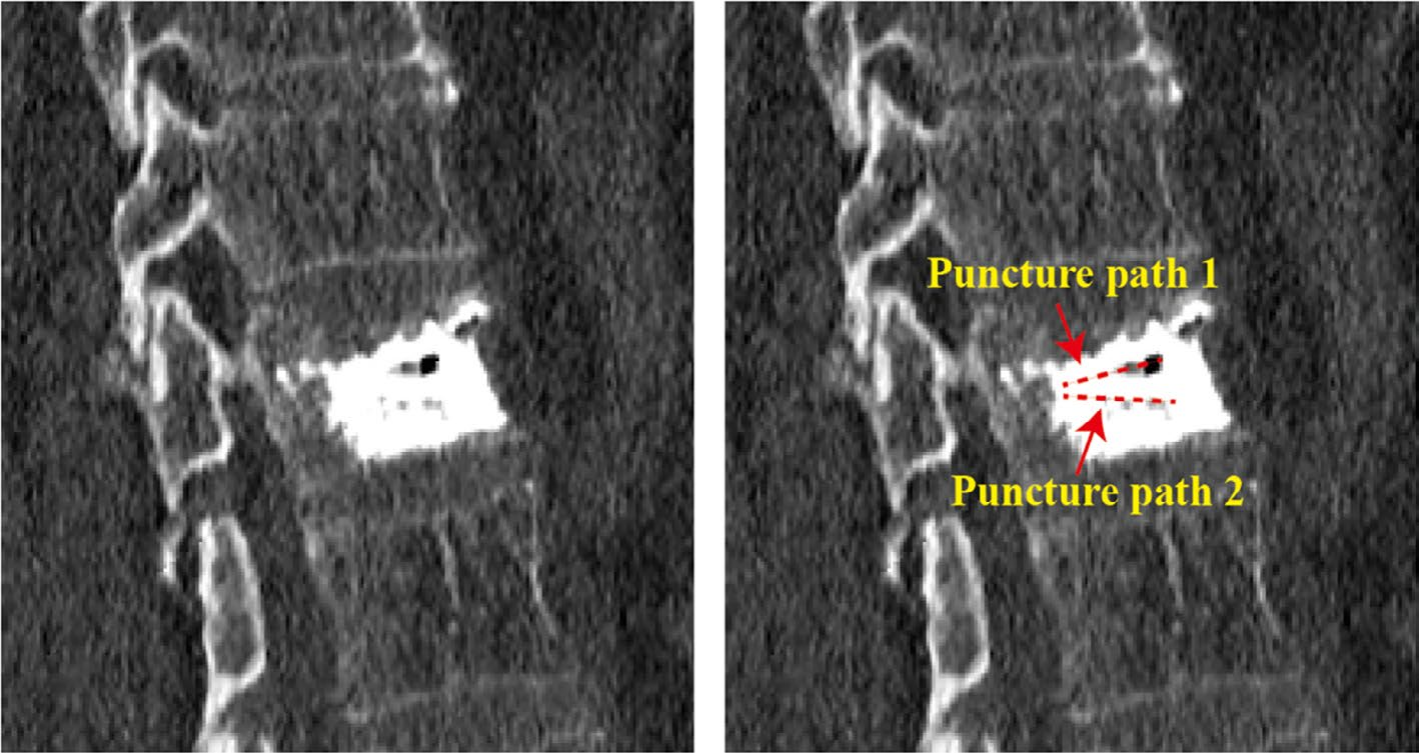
Postoperative CT sagittal images of unilateral dual‐plane puncture showing the original puncture path and the adjusted puncture path.
